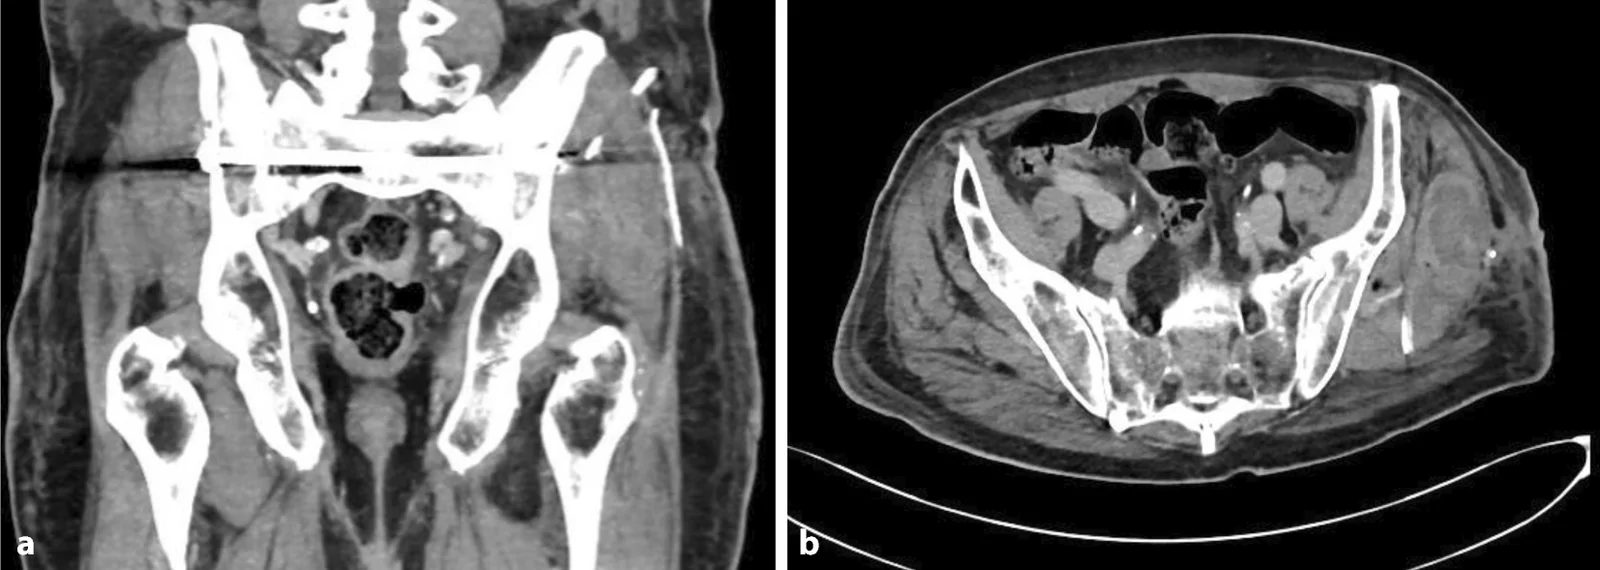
Postoperative kontrastmittelgestützte Becken-Bein-CT-Angiographie ohne Nachweis einer aktiven Blutung im Bereich des ehemaligen Pseudoaneurysmas. Das Hämatom zeigte eine regredierte Größe; einliegende Drainagen (a koronar, b axial)
